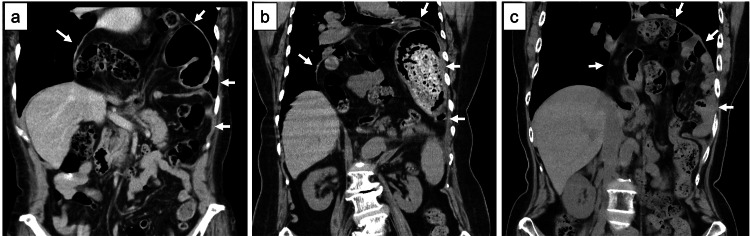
Preoperative computed tomography images of the three representative cases showing large hiatus lengths and hernia sac areas (a) Case 1. (b) Case 2. (c) Case 3. White arrows indicate the margins of the esophageal hiatus and the extent of the hernia sac.
